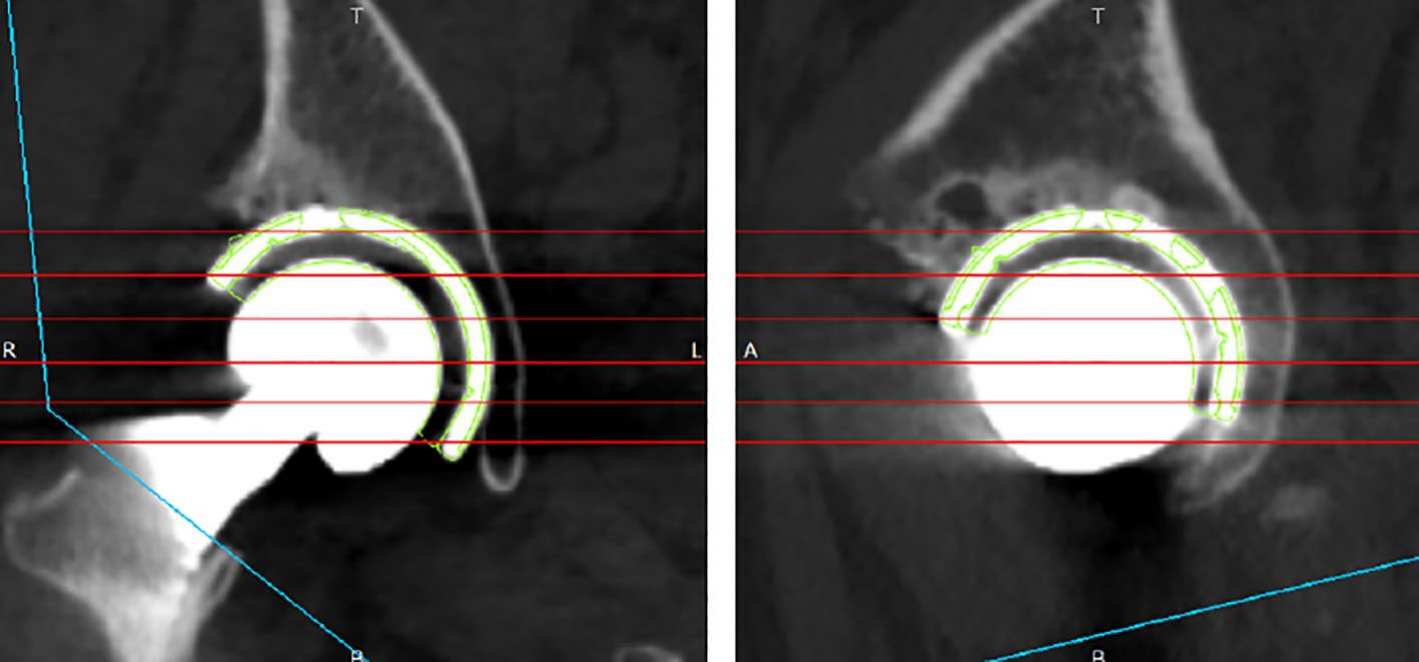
Measurement of radiographic inclination (RI) and radiographic anteversion (RA) using the 3-dimensional digital templating system (ZedHip).
